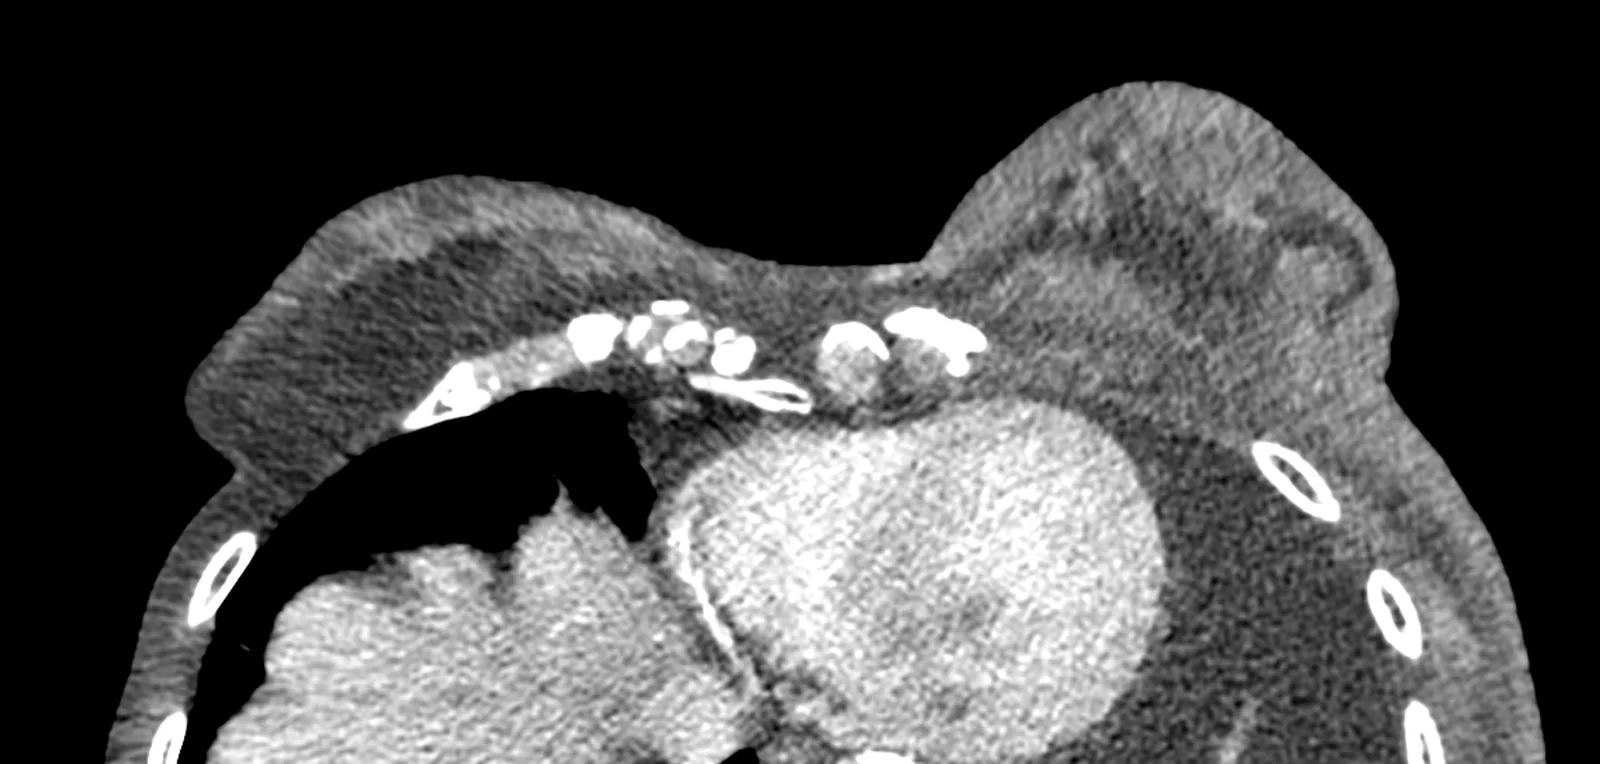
Unlabelled contrast-enhanced CT scan images (partial axial view) of the chest for better appreciation of the abnormalities. CT: computed tomography
